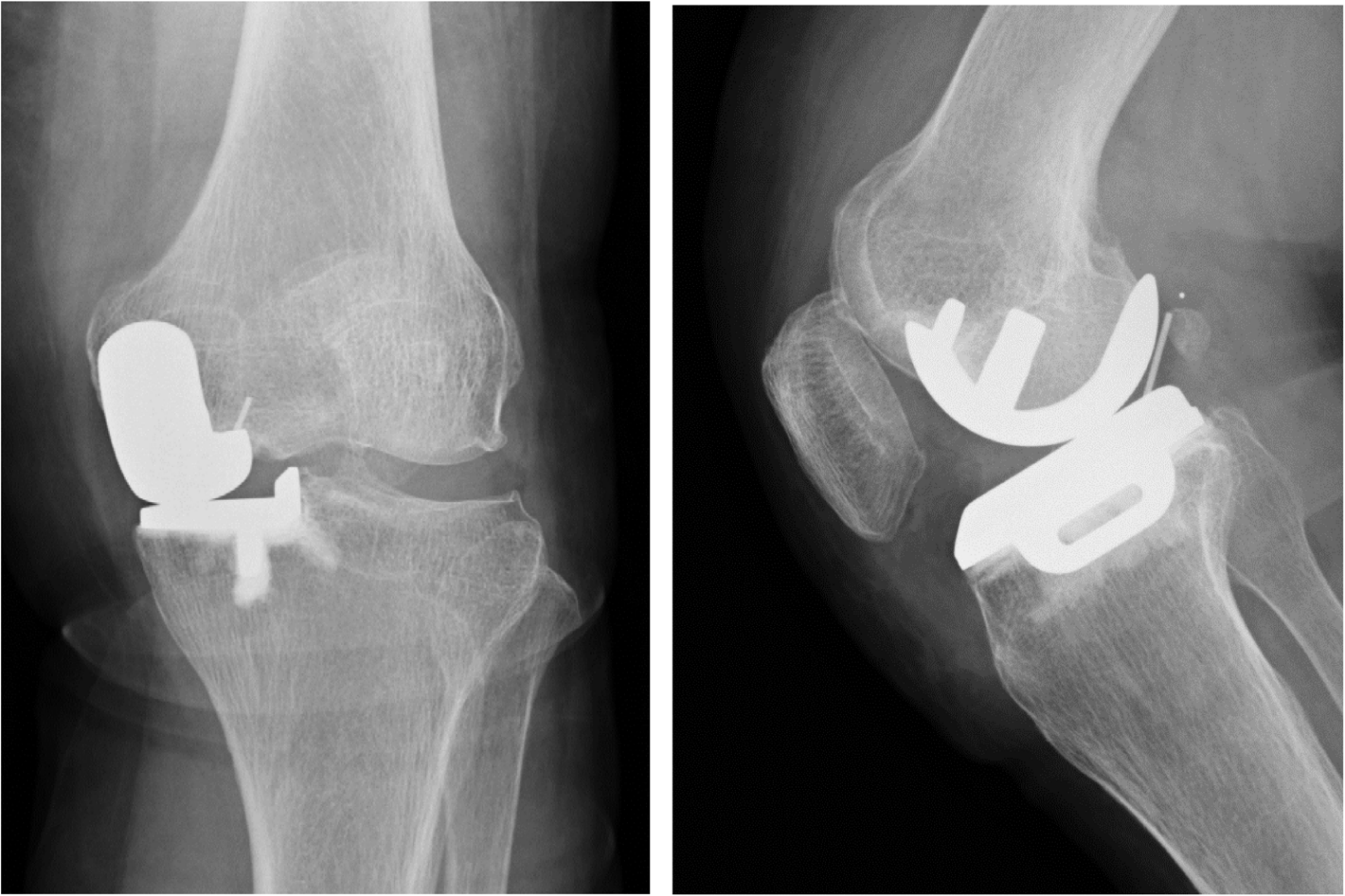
Radiographs of case 1 showing the bearing posterior dislocation
